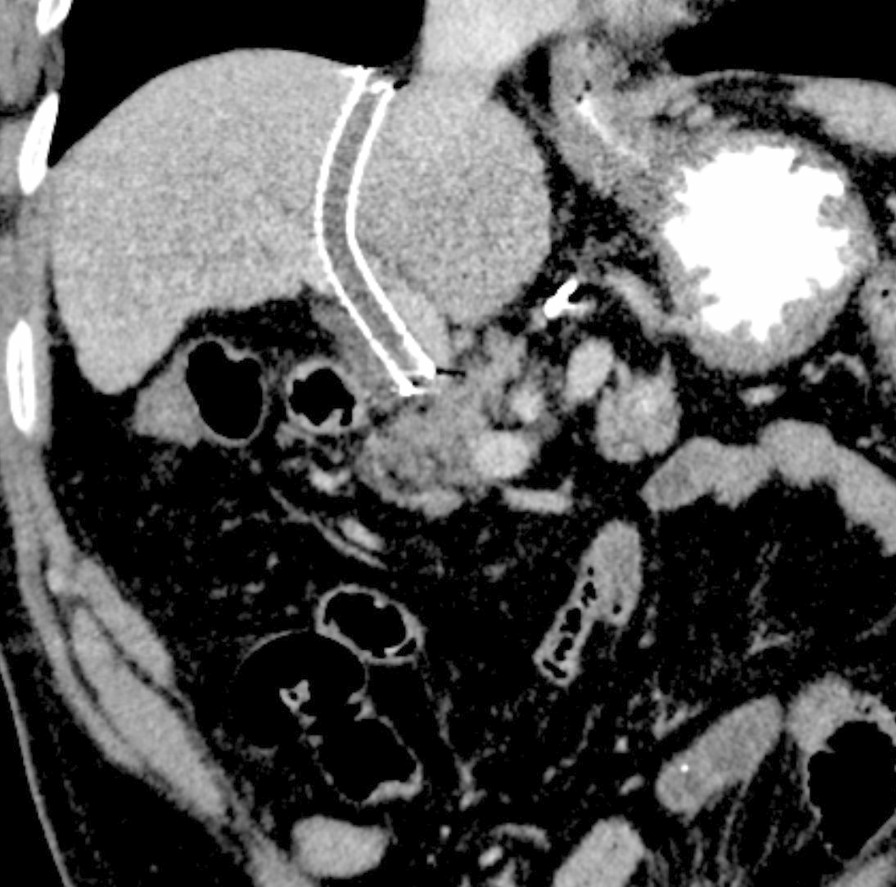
Abdominal CT reconstruction shows tubular high-density shadow in the right branch of the portal vein that passes through the liver parenchyma to the proximal end of the inferior vena cava with low-density shadow in the lumen and no contrast agent filling
